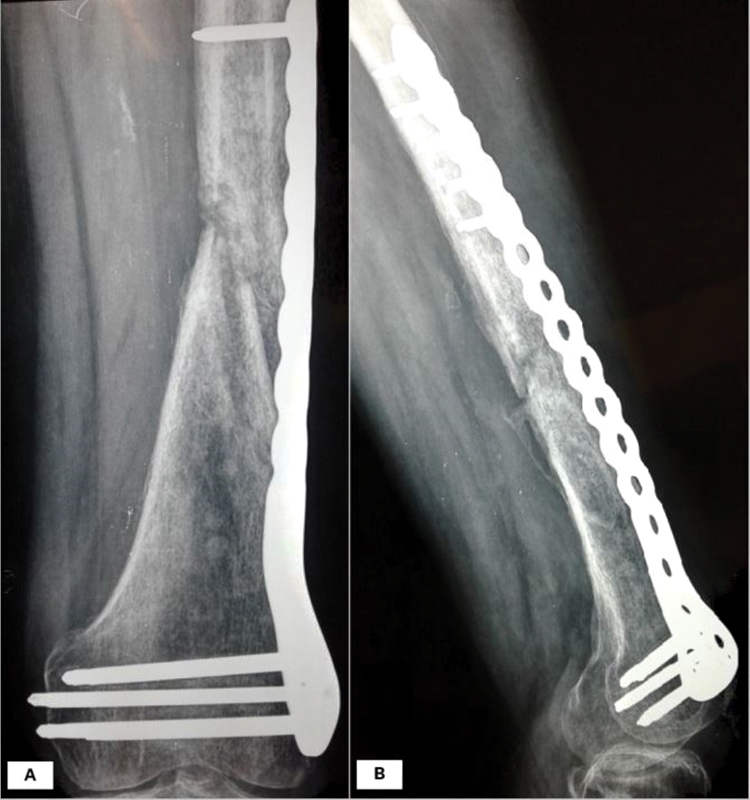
Radiographic image of the fracture following implantation of antibiotic-impregnated cement and distal femur plating system.
